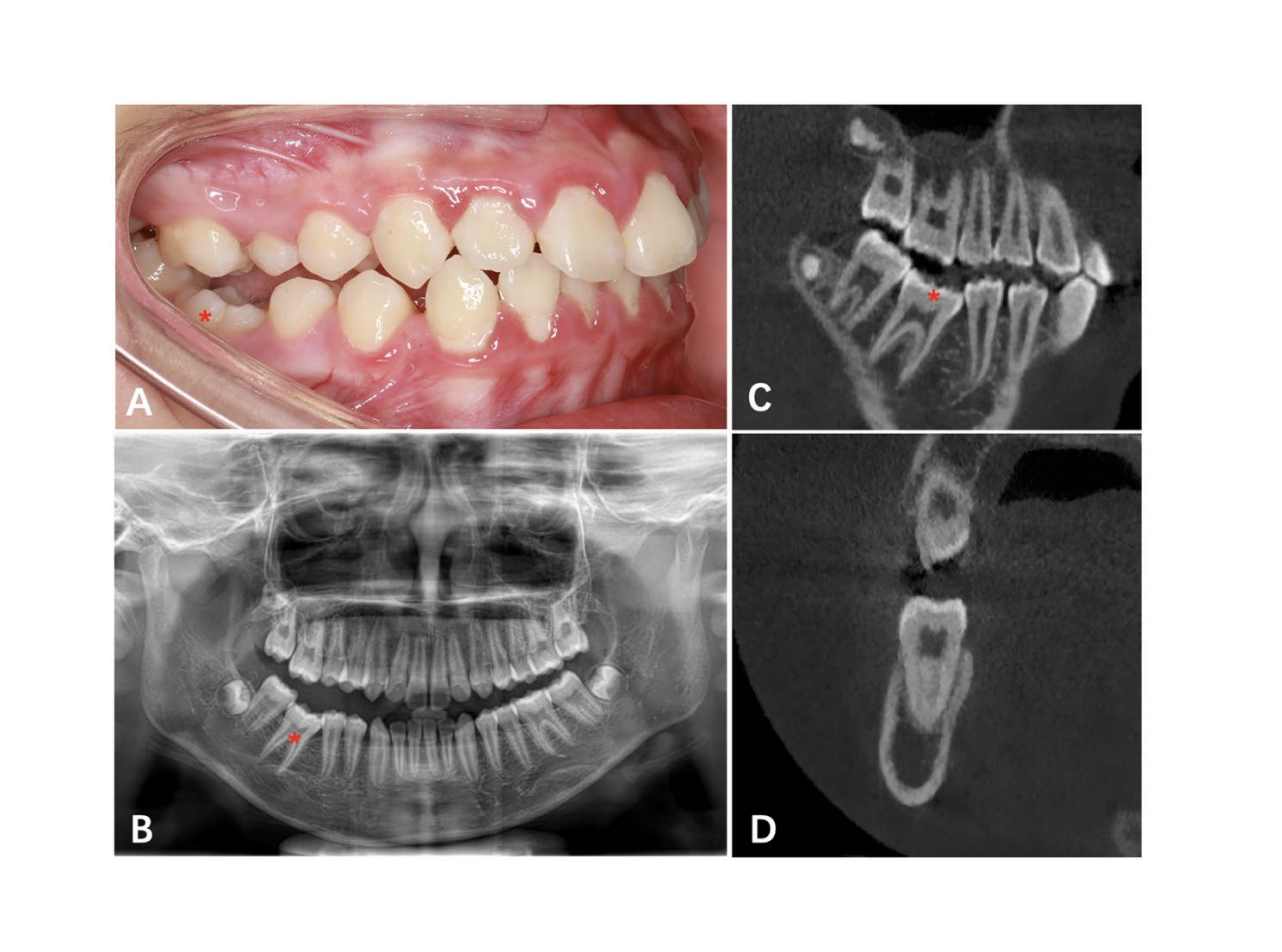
Clinical and radiographic information of the proband’ bother. (A, intraoral left view. B, panoramic radiograph. C, sagittal image of #46. D, coronal image of #46. “*” represent the PFE involved tooth. )
